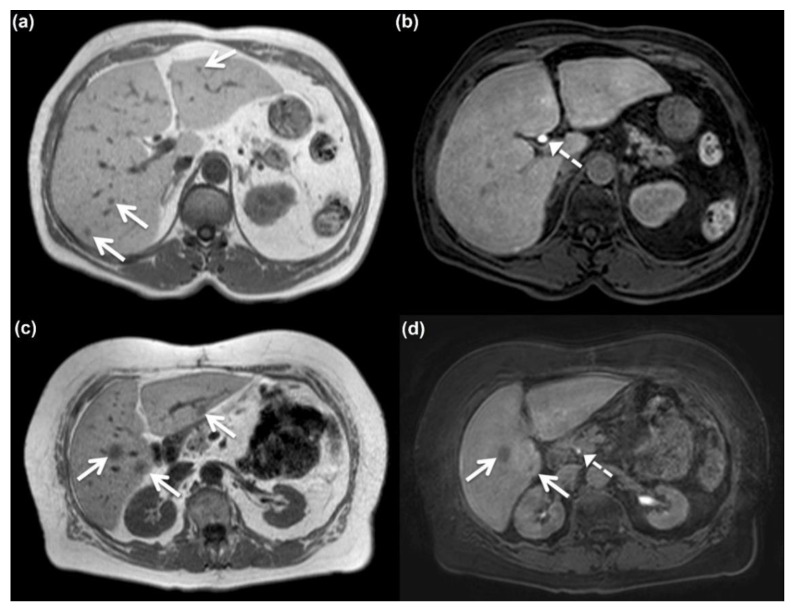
Patient 1 (a,b) and patient 2 (c,d), both with fatty liver and metastatic lesions (arrows) seen on unenhanced T1-weighted non-fat-saturated images (a,c) but only partially positive on the corresponding hepatobiliary phase images (b,d) acquired after gadoxetic acid administration. Note biliary excretion of the contrast agent (broken arrow).
